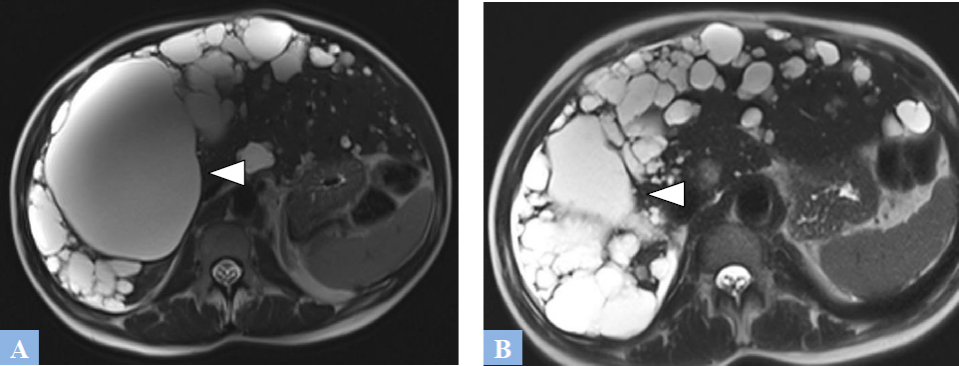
LD in a symptomatic 44-year-old female patient. A. T2-weighted MR image in the axial plane. Predominant cyst in the right liver (arrowhead). B. T2-weighted MR image in the axial plane, follow-up 3 months after percutaneous alcohol ablation of the predominant cyst (arrowhead).
